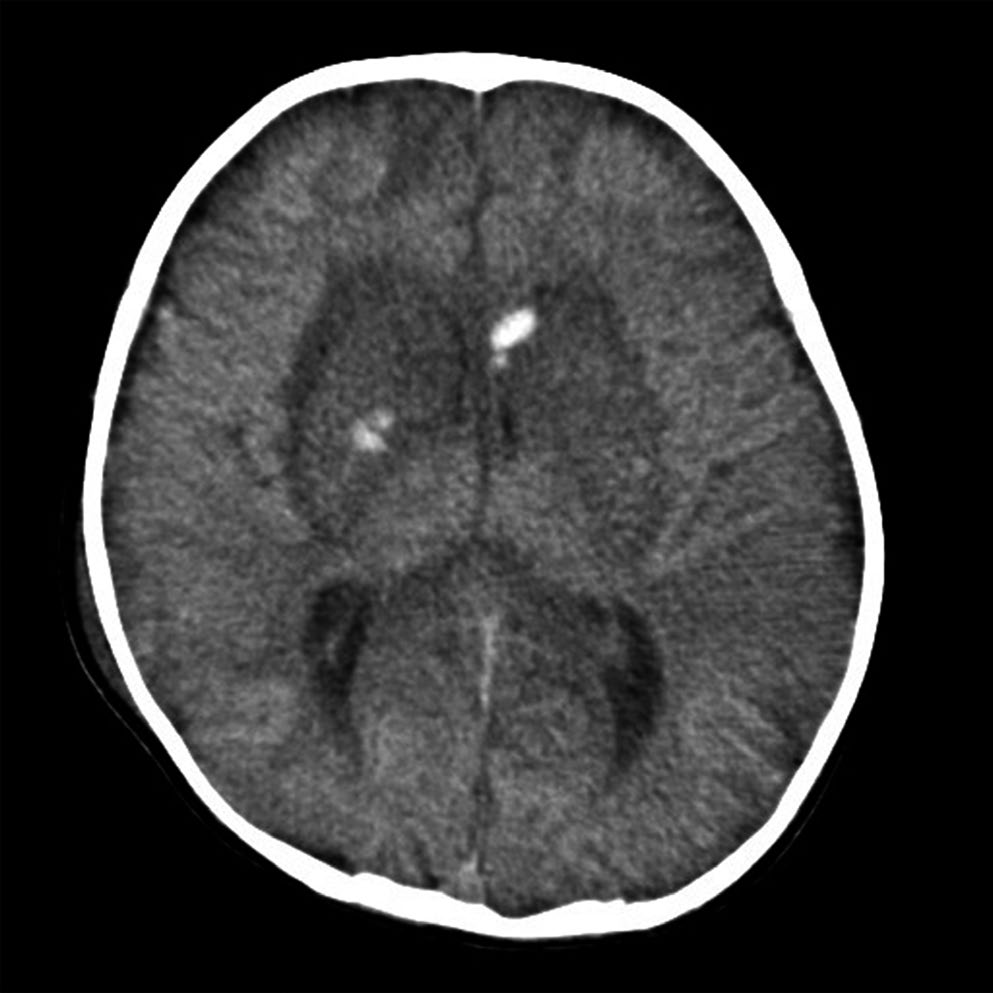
Computed tomography performed 2 wks after admission showing overall high‐density lesions in the cerebral cortex and hemorrhage in the bilateral basal ganglia (arrows)
